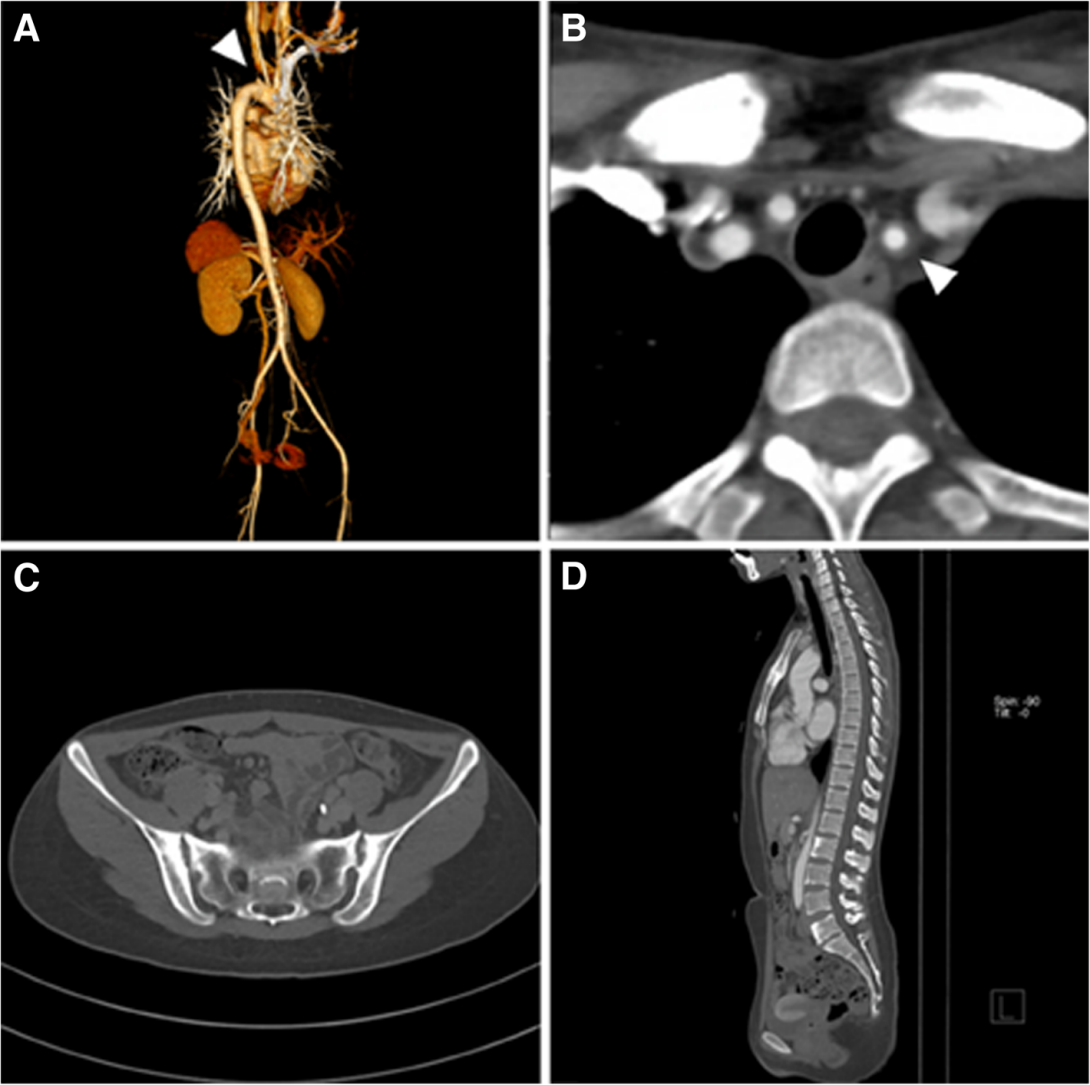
Computed tomography (CT) images of Takayasu arteritis (TAK) patient who exhibited sacroiliitis as an extravascular manifestation. Female patient, 29 years old, negative for HLA-B27. a Complete occlusion in left subclavian artery (arrowhead). b Concentric wall thickening in left common carotid artery (arrowhead). c Sclerosis in both sacroiliac joints and erosion with joint space widening suggesting grade III sacroiliitis. d Vertebral corner inflammations, especially in lumbar spines (L2 and L5)
